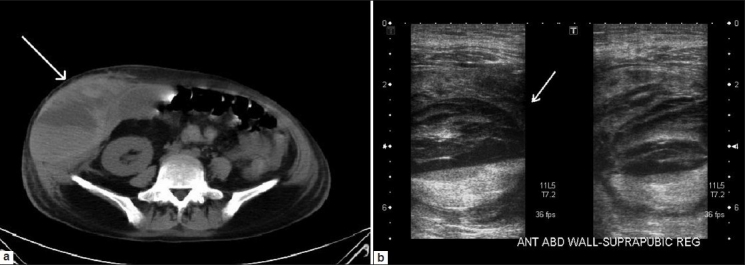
Ultrasound image (a) and computed tomography image (b) showing the hematoma (arrows) within the abdominal wall overlying the graft kidney
